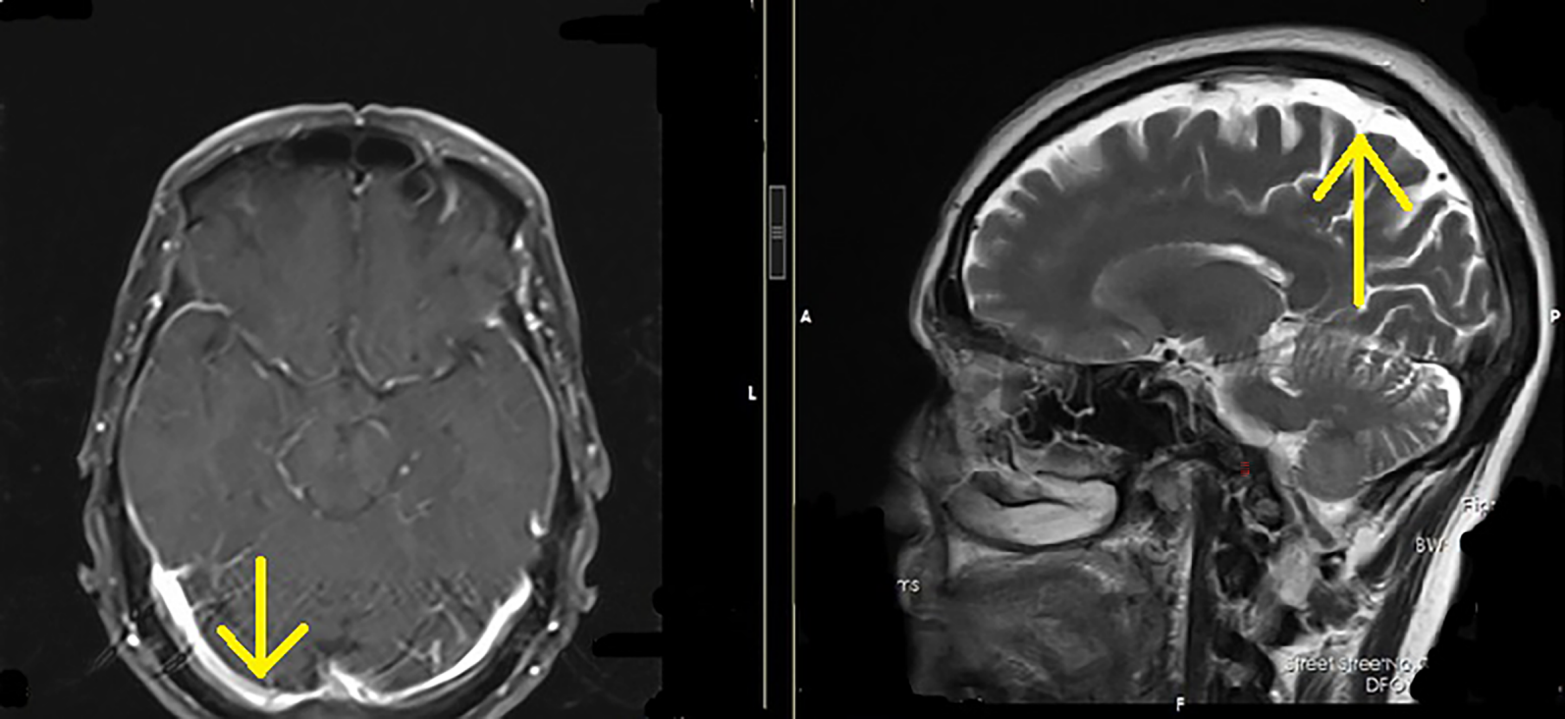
Post‐contrast T1 weighted sequences of the brain MRI at the time of diagnosis of leptomeningeal involvement (April 2022): (A) Axial plane at the level of midbrain and (B) Sagittal planes shows abnormal enhancement in dura (yellow arrows).
